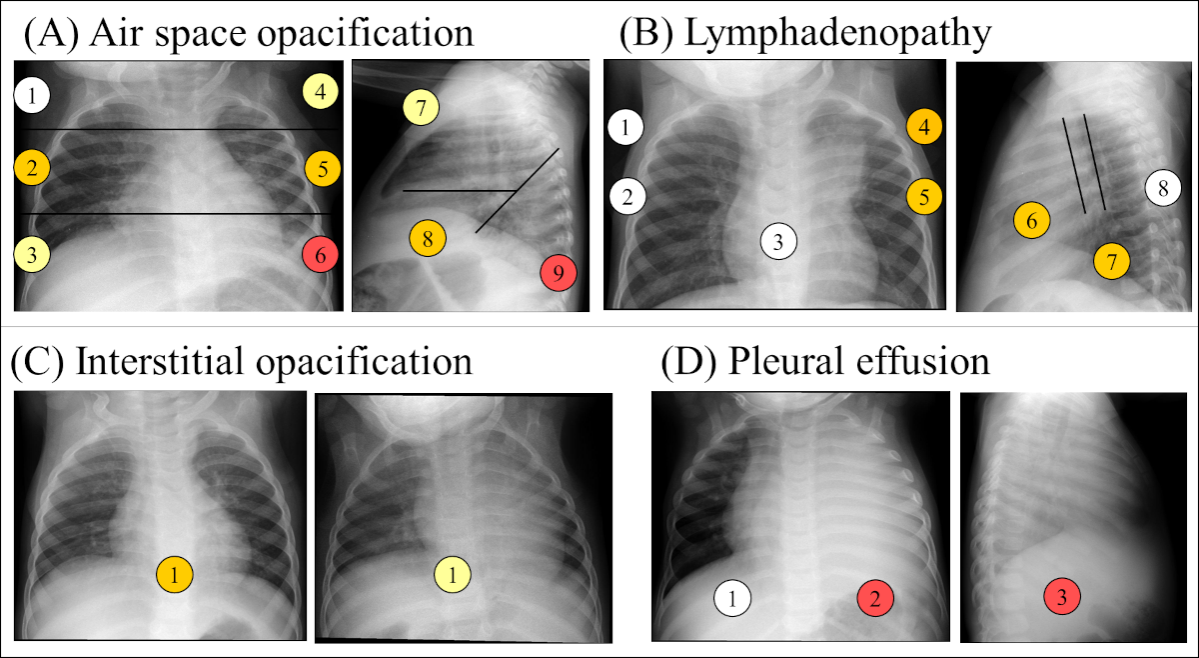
Example of evaluations of findings in different studies. The locations of the findings are defined in Figure 3. The color of the locations represents the number of evaluators that identified the presence of the finding in that location, being 0 evaluators for white, 1 evaluator for yellow, 2 evaluators for orange, and 3 evaluators for red. (A) Presence of air space opacification in the anteroposterior (AP) and lateral chest x-ray (CXR) views of an examination of a female patient of 11 months classified as unconfirmed tuberculosis (TB) and as suggestive of TB by 1 out of the 3 evaluators. (B) Presence of lymphadenopathy in the AP and lateral CXR views of an examination of a female patient of 11 months classified as confirmed TB and as suggestive of TB by the 3 evaluators. (C) Presence of interstitial opacification on AP CXR views of 2 studies, the one on the left is from a male patient of 1 year and 4 months of age. Both studies were classified as unconfirmed TB and not suggestive of TB. The AP view on the right corresponds to a patient of female of 11 months of age. The examination was classified as unlikely TB and 1 out of 3 evaluators assessed it as confirmed TB. (D) Presence of pleural effusion in the AP and lateral view of an examination of a male of 2 years and 2 months of age classified as confirmed TB and evaluated as suggestive of TB by the 3 evaluators.
